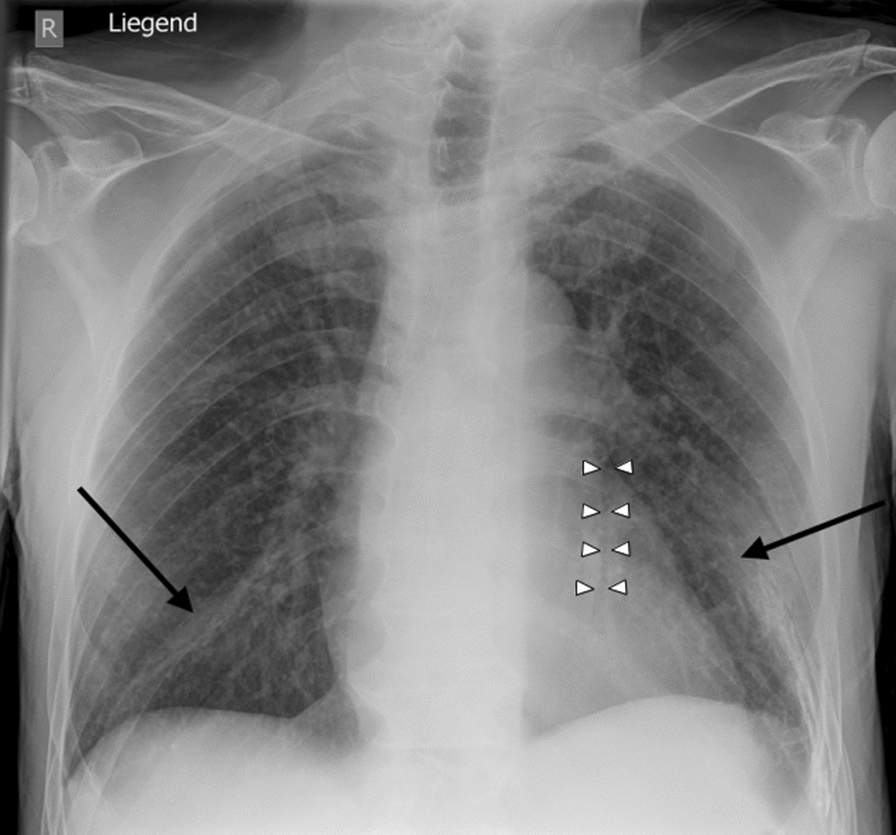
Chest X-ray (supine) from day 1 showing only isolated areas of decreased transparency (black arrows) and a positive retrocardial air bronchogram (white arrows)
